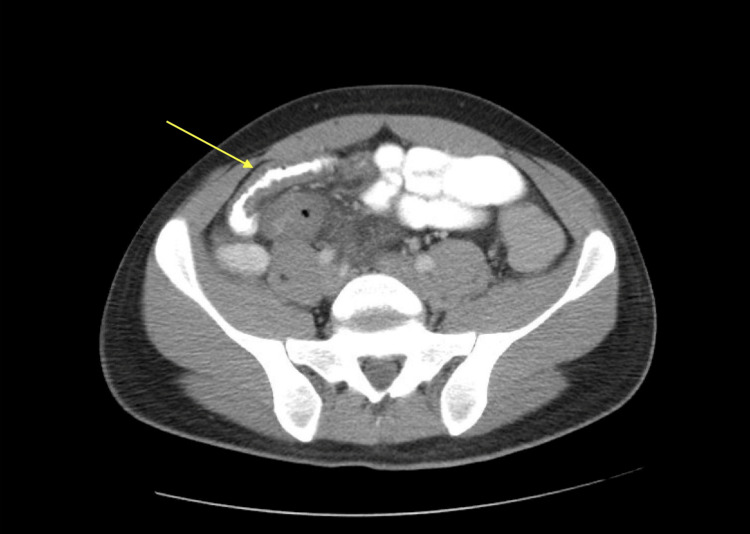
CT scan of the abdomen and pelvis with and without contrast The pointed arrow represents inflammation of the terminal ileum and segment of the distal ileum with prominent wall thickening and mural edema.
